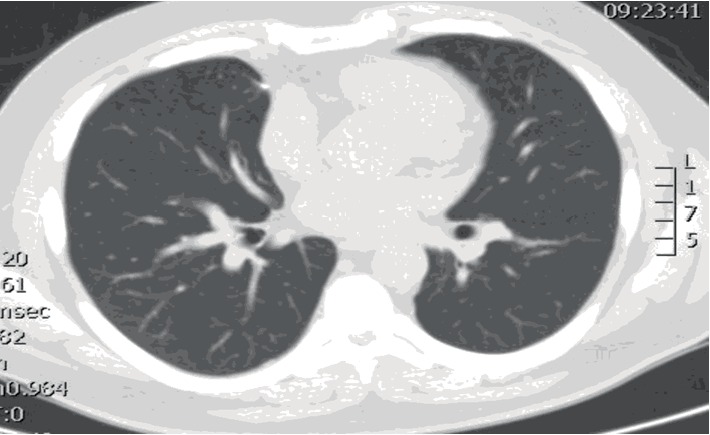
CT chest (axial view) demonstrating complete resolution of lung infiltrates, two months post discharge from the hospital.
